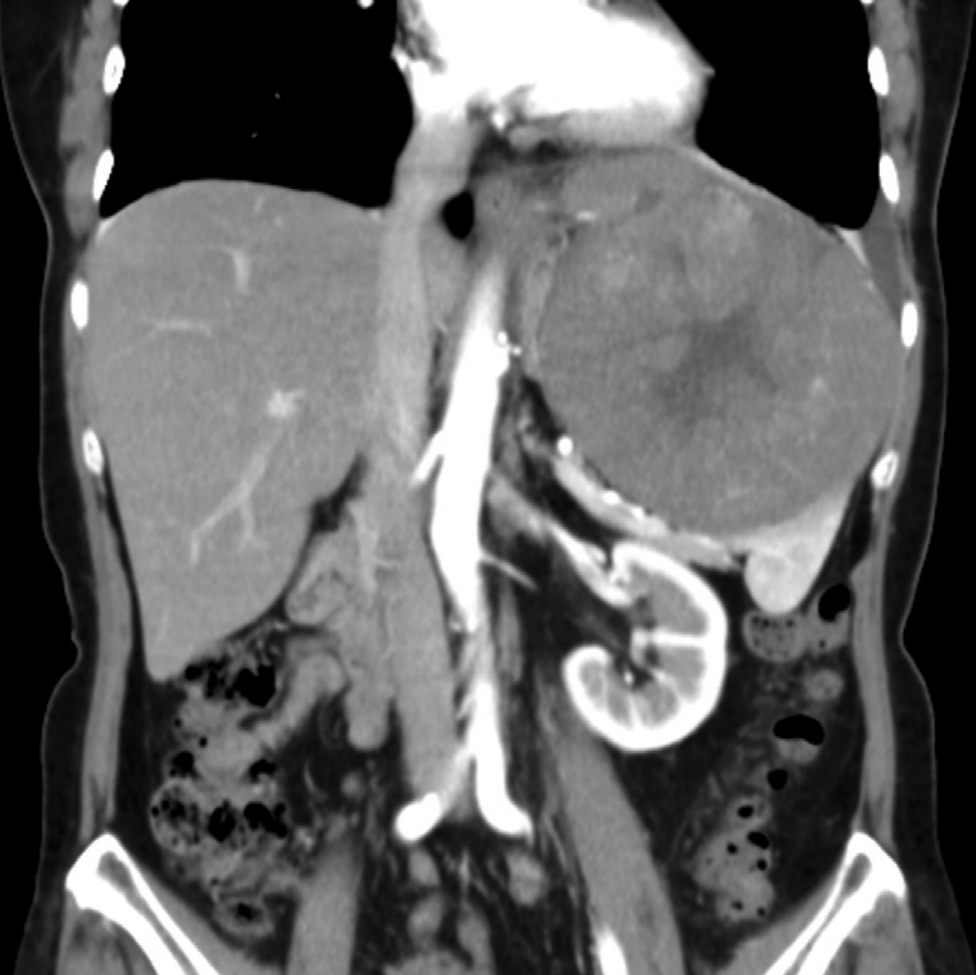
Computed tomography showed a 14 × 13 × 12 cm tumor in the upper left region of the abdominal cavity that was unevenly enhanced in the arterial phase.
